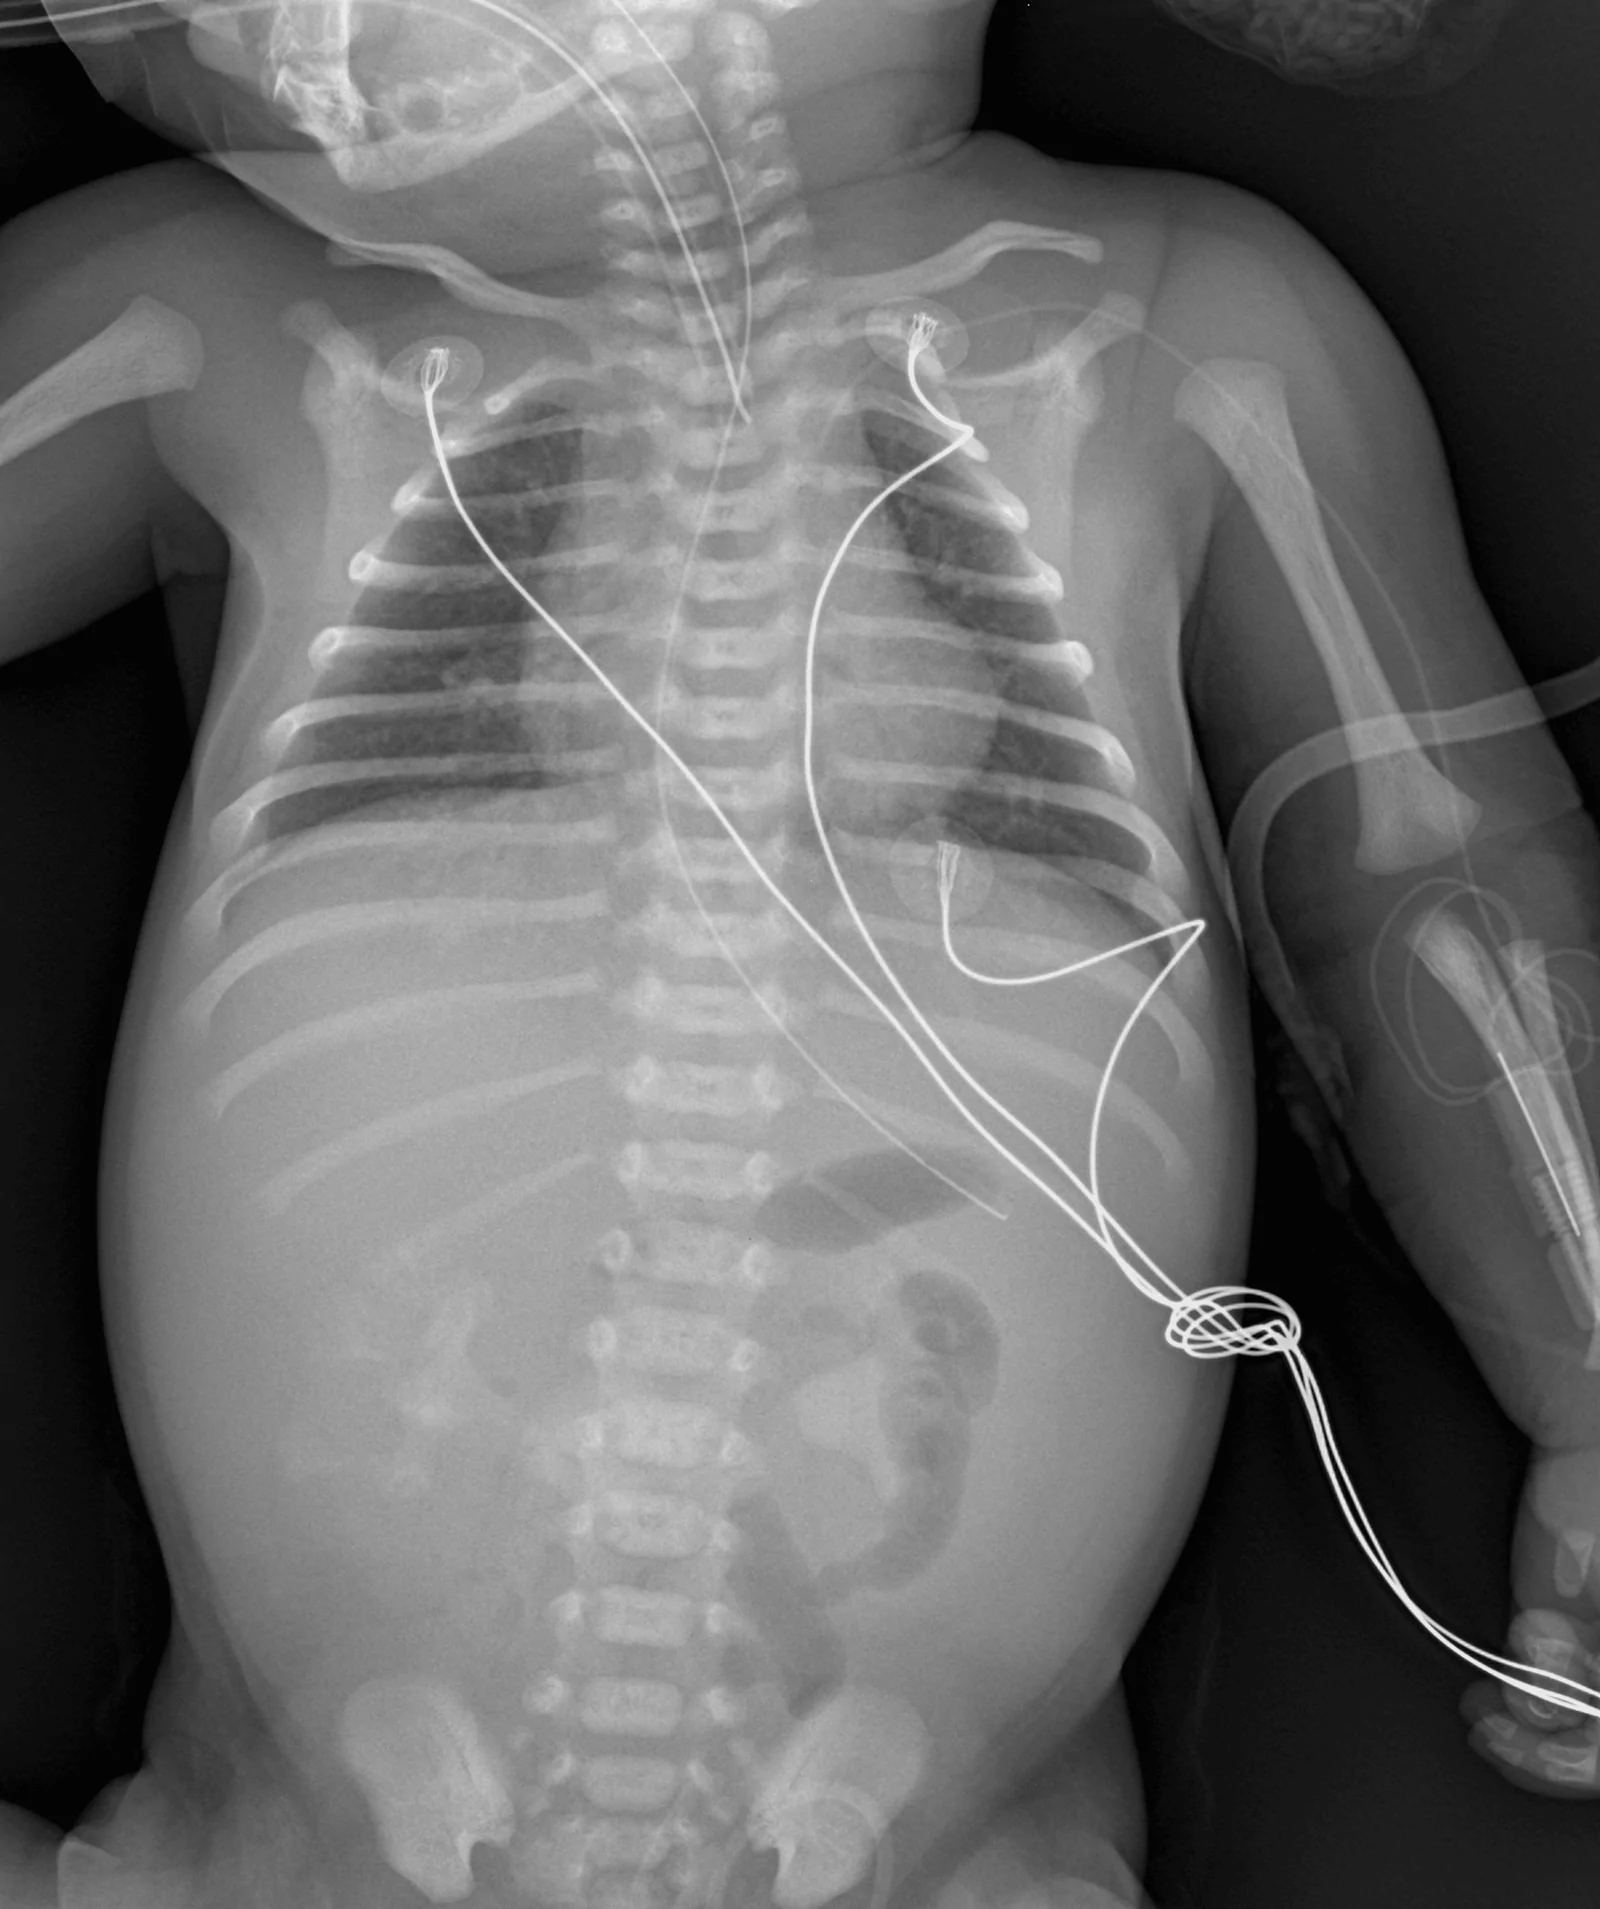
Abdominal anterior-posterior radiograph showing little visible air in the bowel loops.
